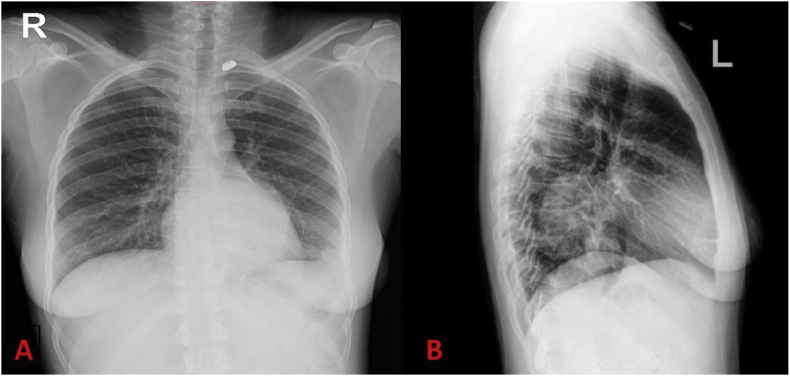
A. Antero-lateral view, B. Lateral view - shows bilateral hilar lymphadenopathy and bilateral infiltrates on a chest x-ray.
